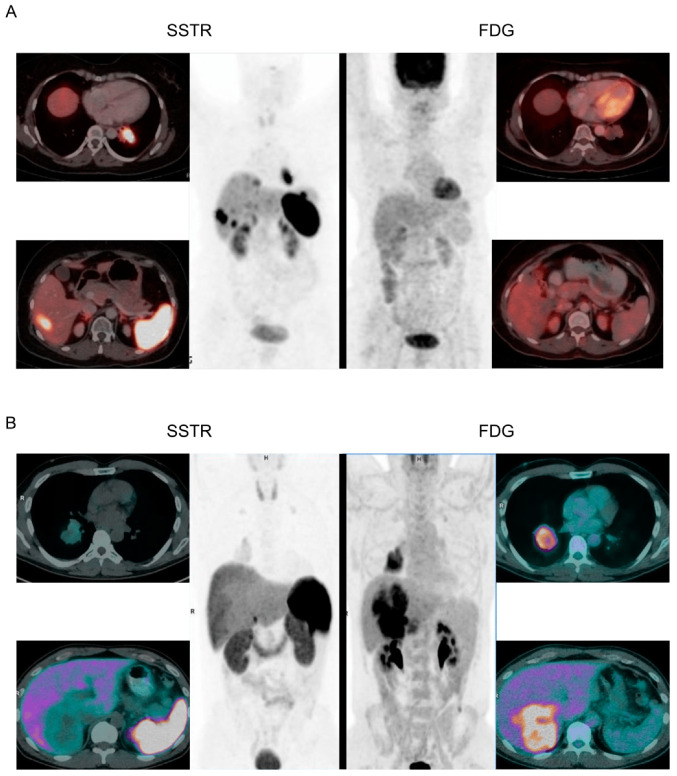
Functional scans of L-NETs and L-NECs. (A): A patient with L-NET was scanned with both a somatostatin analog (SSTR) scan and a glucose analog scan (FDG). The SSTR scan shows high uptake of somatostatin in the primary tumor and in a liver metastasis, while the FDG scan shows no uptake of FDG in the primary tumor and does not reveal the liver metastasis. (B): A patient with L-NEC was scanned with both an SSTR scan and an FDG scan. The FDG scan shows high uptake of FDG in the primary tumor and in a large conglomerate of liver metastases, while the SSTR scan shows no uptake of somatostatin in the primary tumor and does not reveal the liver metastases. Adapted from Purandara et al. and Chan et al. [32,33].
